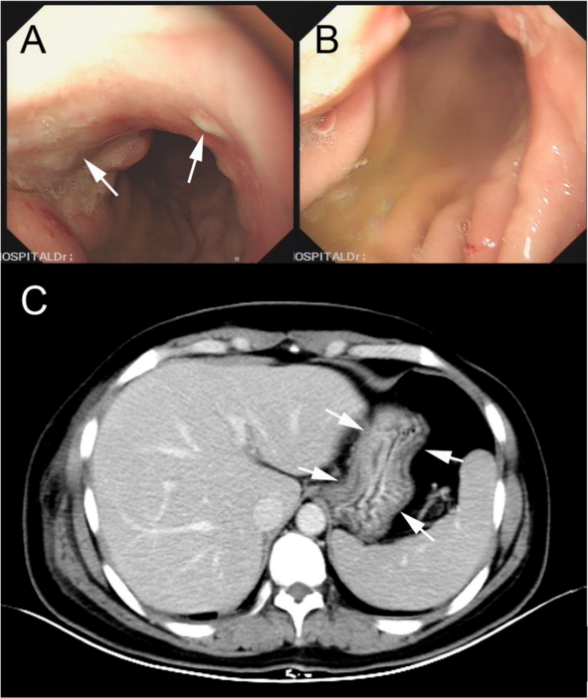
Gastric endoscopy and enhanced abdominal CT scan. Gastric endoscopy showed an ulcerative mass in the gastric body (arrows) (A,B). Enhanced abdominal CT scan revealed a circumferential mural thickening of the gastric body wall (arrows) (C).
